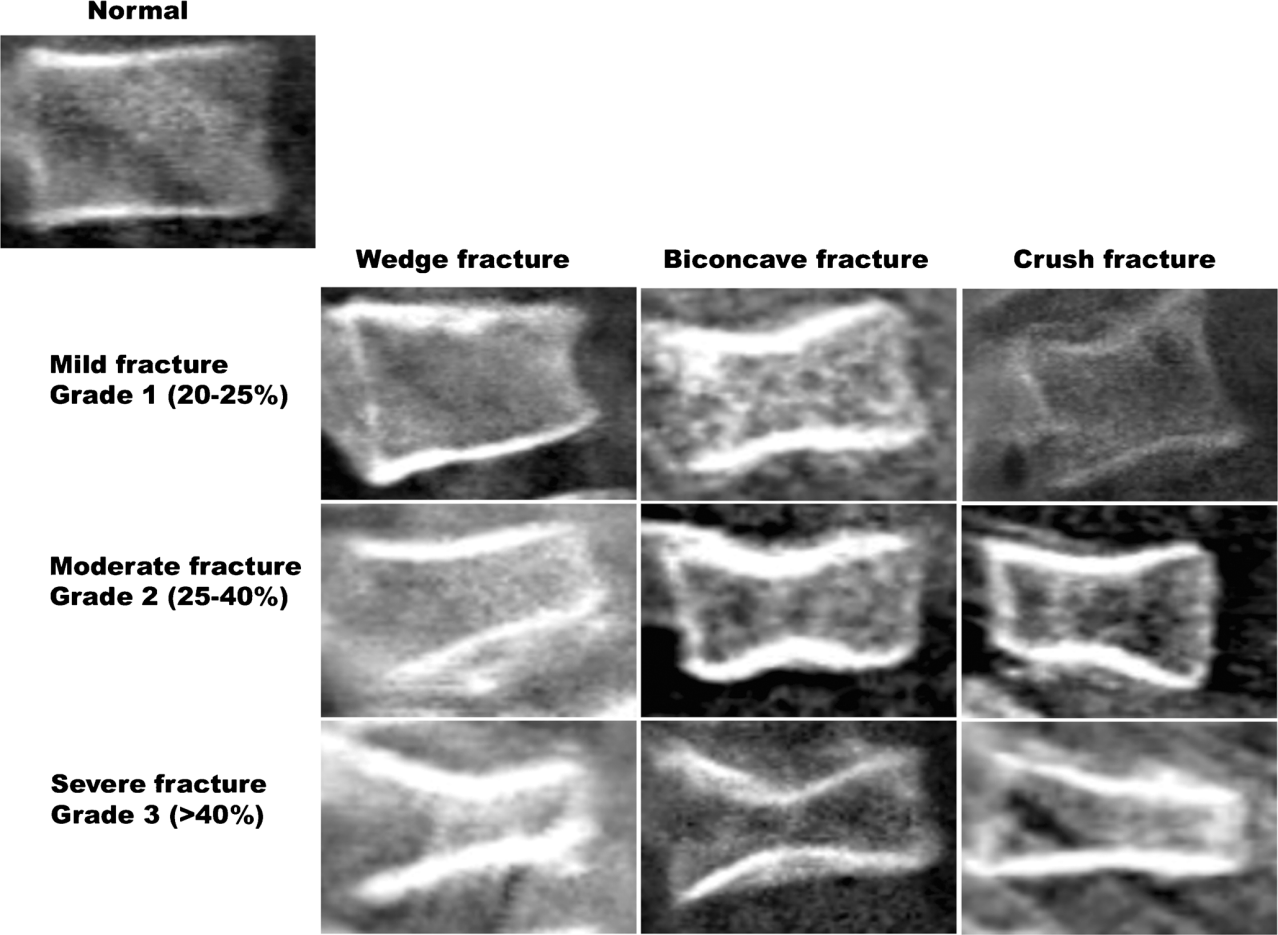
Selected lateral spine dual energy x-ray absorptiometry scans from a series of patients demonstrate the semiquantitative visual grading system of Genant et al. [10]
